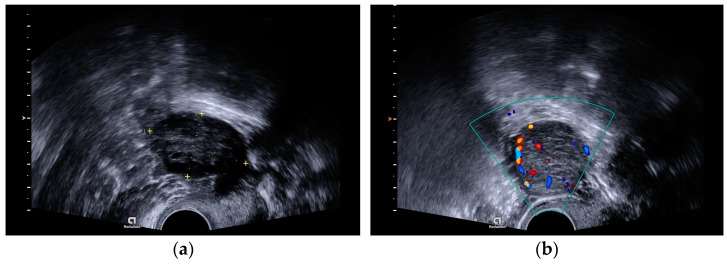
(a) A transvaginal ultrasound scan showing a polycystic structured ovary. (b) A transvaginal ultrasound scan showing a left ovary tumor with strong blood flow.
